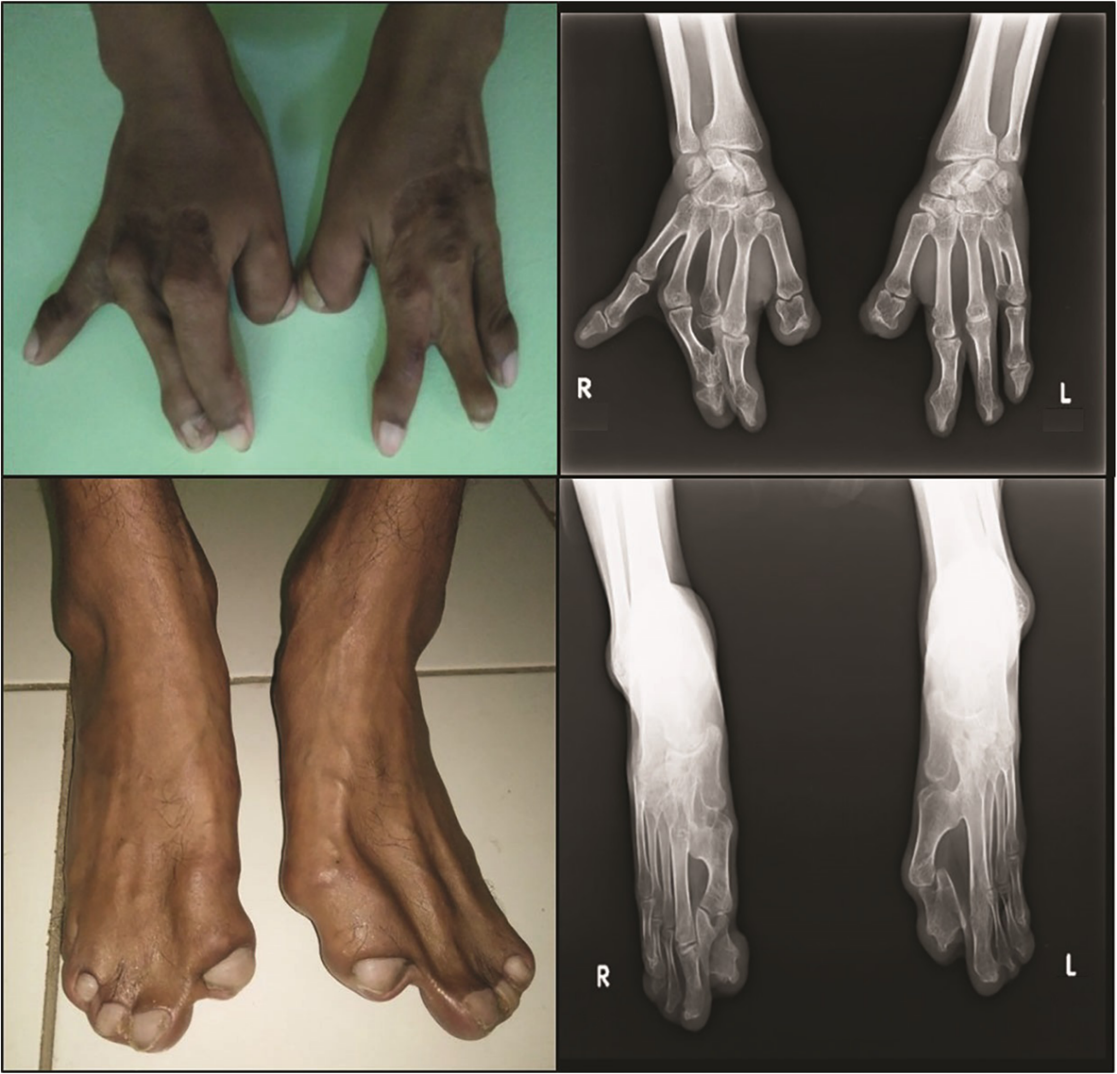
Radiological features of the hands and feet of the patient. Photographs and radiographs showing lack of digit type 2 (fused), symmetrical syndactyly of four digits in both hands (*upper panels*), and type 2 symmetrical syndactyly of five toes in both feet (*lower panels*)
